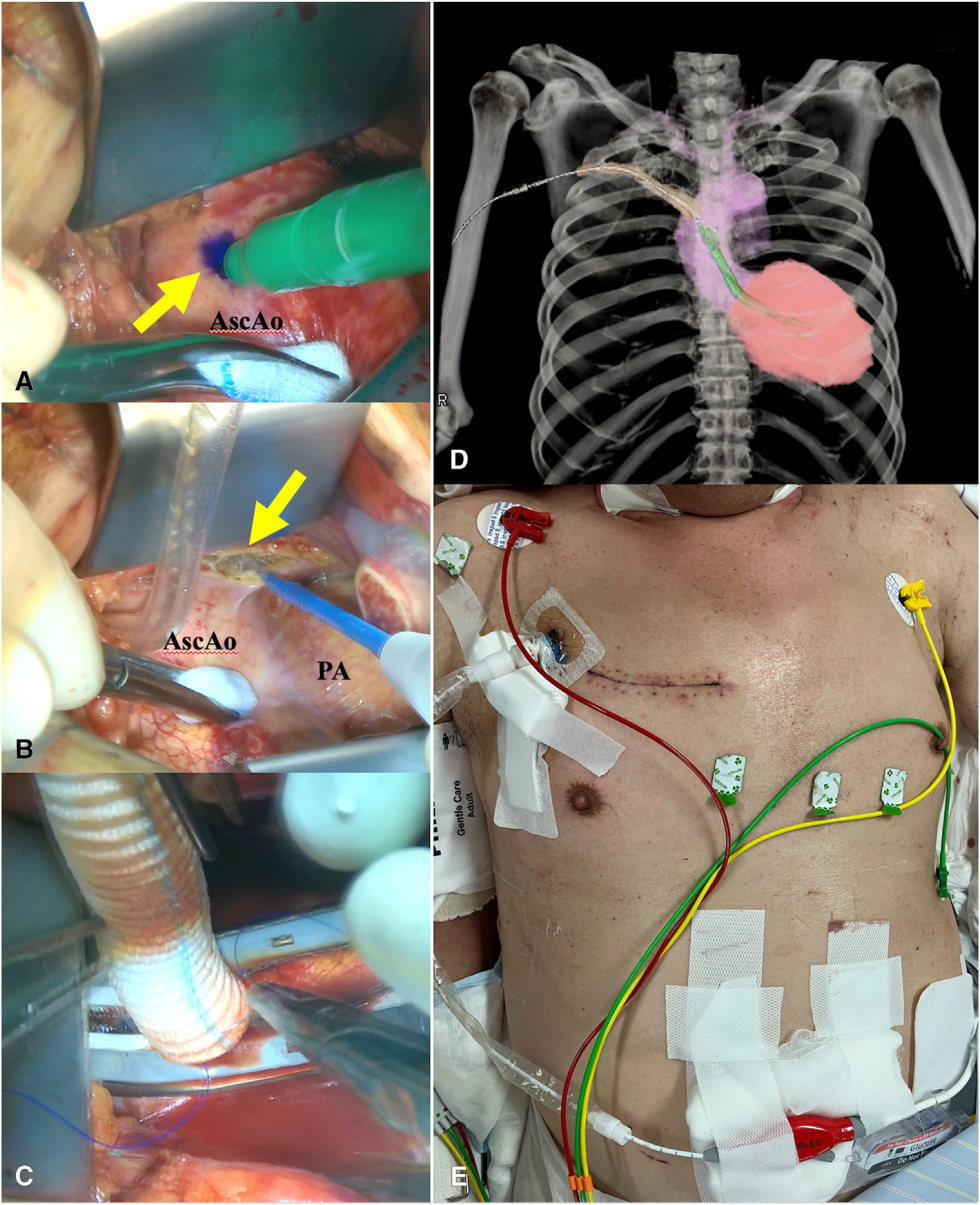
A, *Yellow arrow* shows the anastomosis site 1 cm below the origin of innominate artery. B, *Yellow arrow* shows the resected reflection of pericardium. C, End-to-side anastomosis performed using a partial clamp at the center of the wound. D, Postoperative computed tomography showing adequate position and gentle direction of Impella 5.5 in ascending aorta and left ventricle. E, Impella 5.5 was placed similar to the axillary approach. *AscAo*, Ascending Aorta; *PA*, pulmonary artery.
